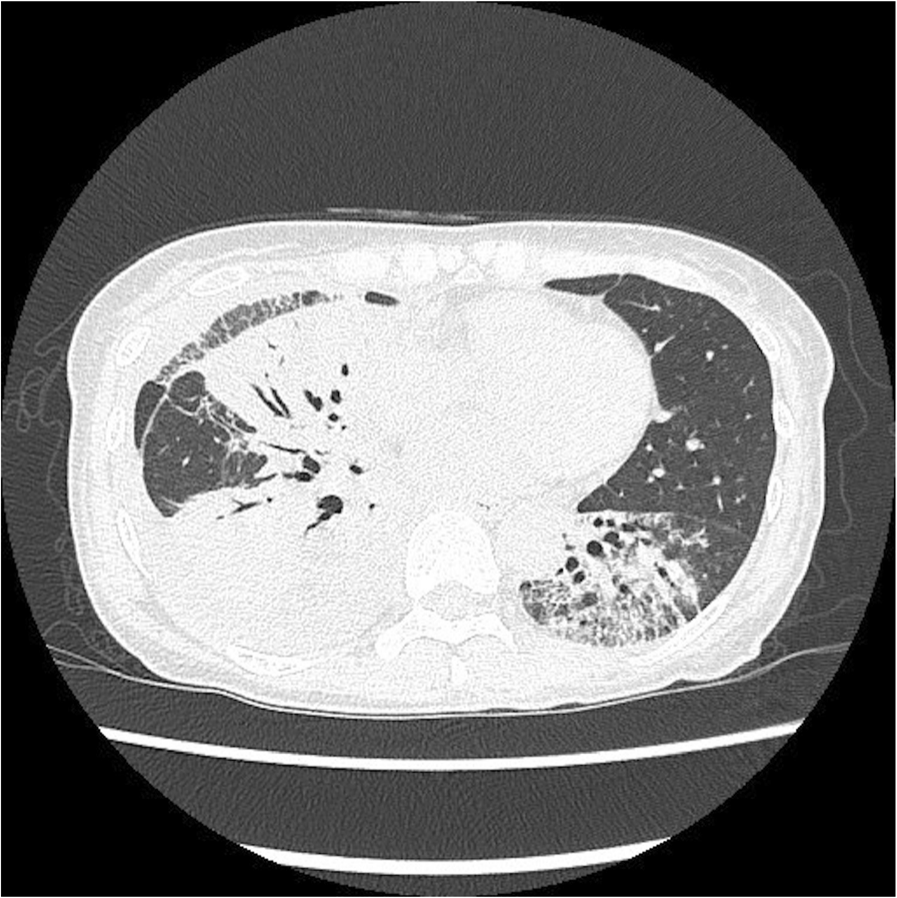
Chest CT image on admission
